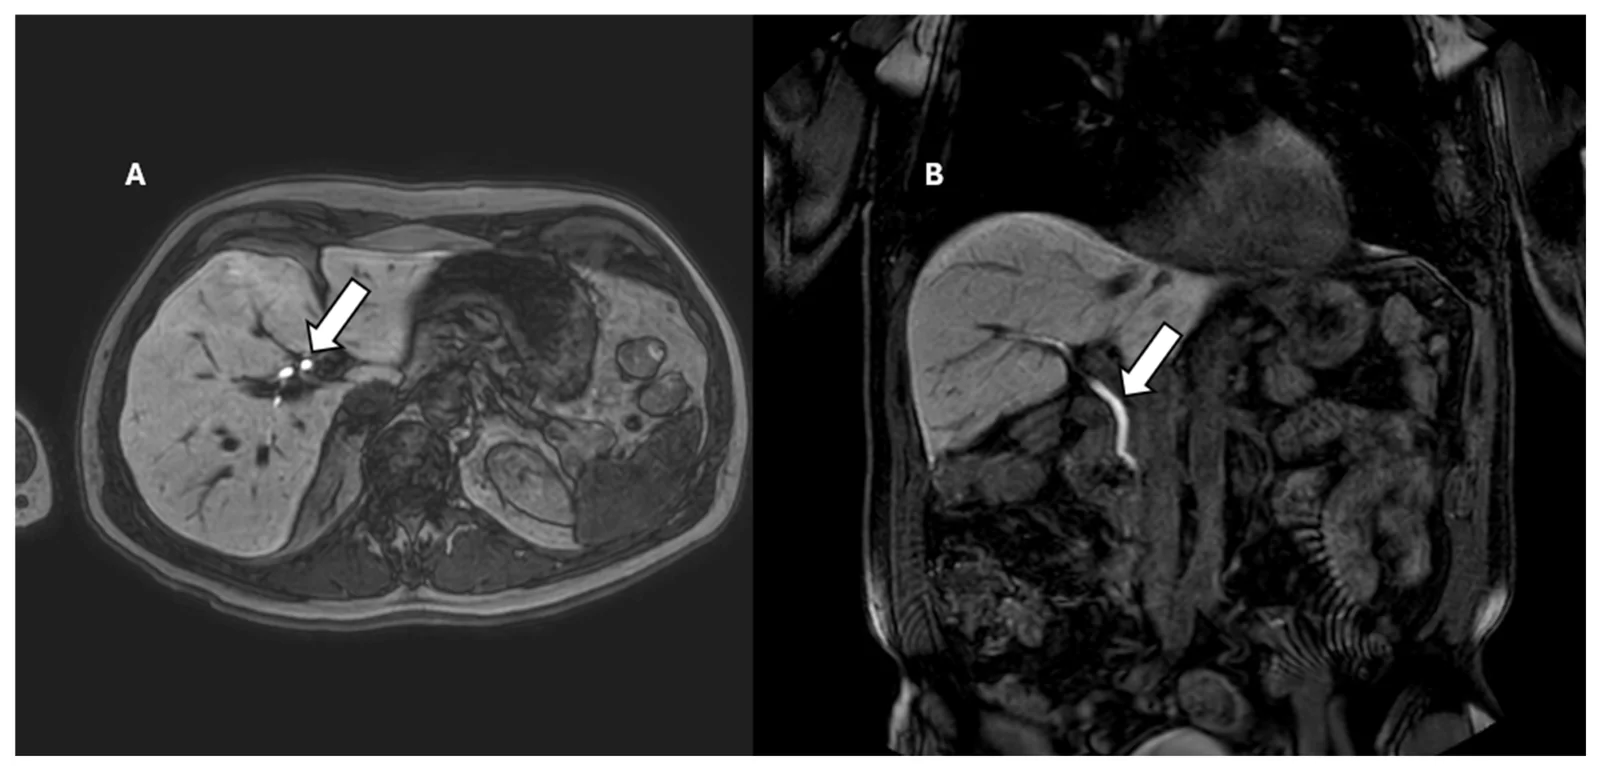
Functional hepatobiliary contrast excretion in a normal liver. (A) Axial and (B) coronal hepatobiliary phase MRI images obtained after Gd-EOB-DTPA administration demonstrate normal hepatocyte uptake and physiological excretion of contrast material into the intrahepatic and extrahepatic biliary tree (arrows).
